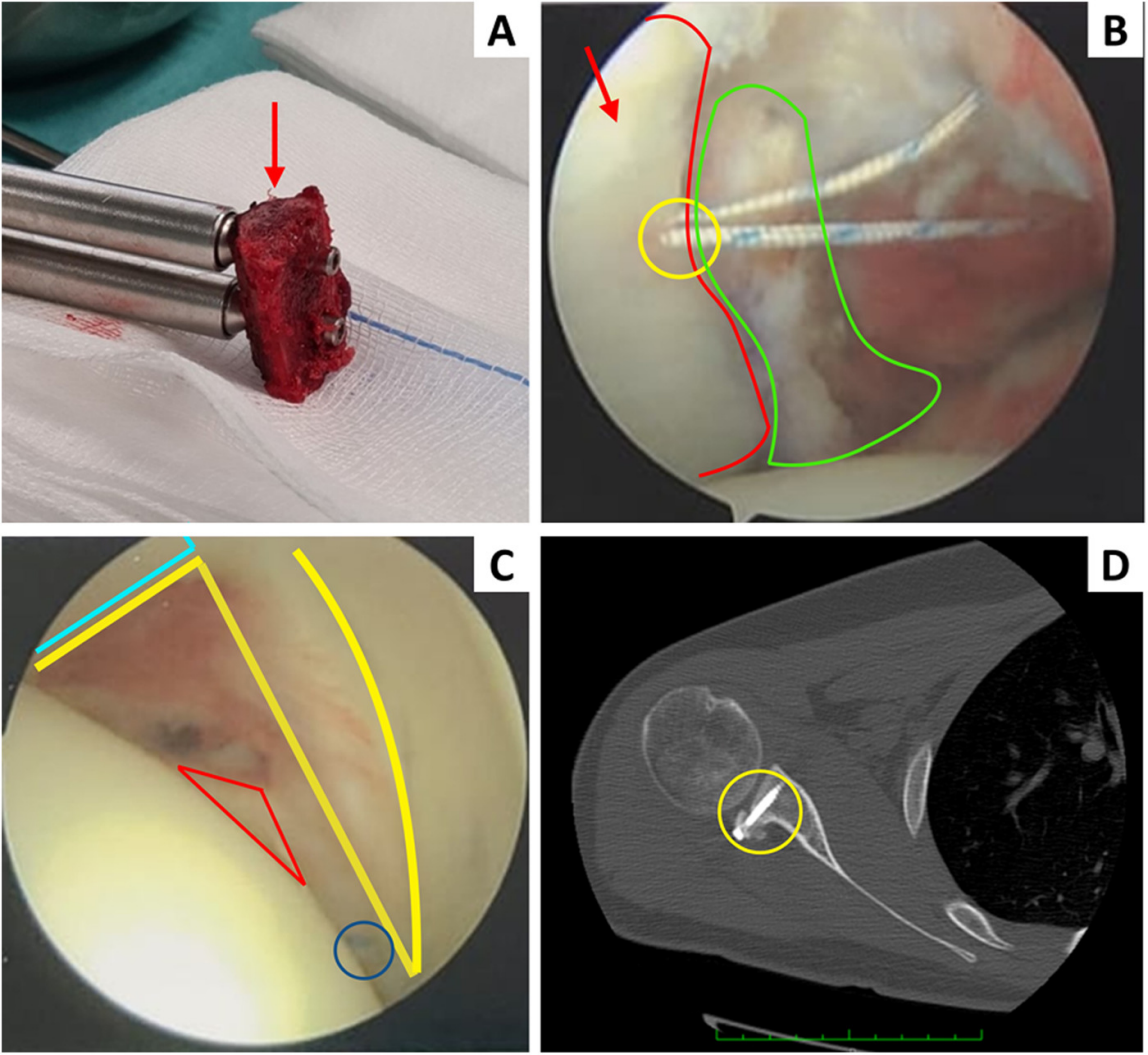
(A) Iliac crest autograft is sourced and ready for reimplantation. The red arrow indicates the iliac crest graft, which was modelled and positioned using the Arthrex®guide for Latarjet surgery. (B) Bankart lesion in the left shoulder from an arthroscopic perspective. The picture provides a frontal view of the posterior part of the left glenohumeral joint. The surgical lip and the posterior capsule will be reattached using a FiberTak® Soft Anchor (Arthrex®) previously inserted into the bone (yellow). The articular surface of the glenohumeral joint is highlighted in red, while the iliac crest graft is shown in green. (C) This is an arthroscopic view of the ASA Technique being performed on the left shoulder. The long head of the biceps brachii is marked in light blue, the anterior part of the glenoid lip is in yellow, the subscapularis muscle following the ASA technique is marked in red, the FiberTak® Soft Anchor (Arthrex®) is circled in dark blue. (D) CT scan of the right shoulder following bone block repair and ASA Technique. Following CT evaluation, the graft (yellow circle) has been positioned posteriorly to the glenoid using two cannulated Arthrex®screws.
